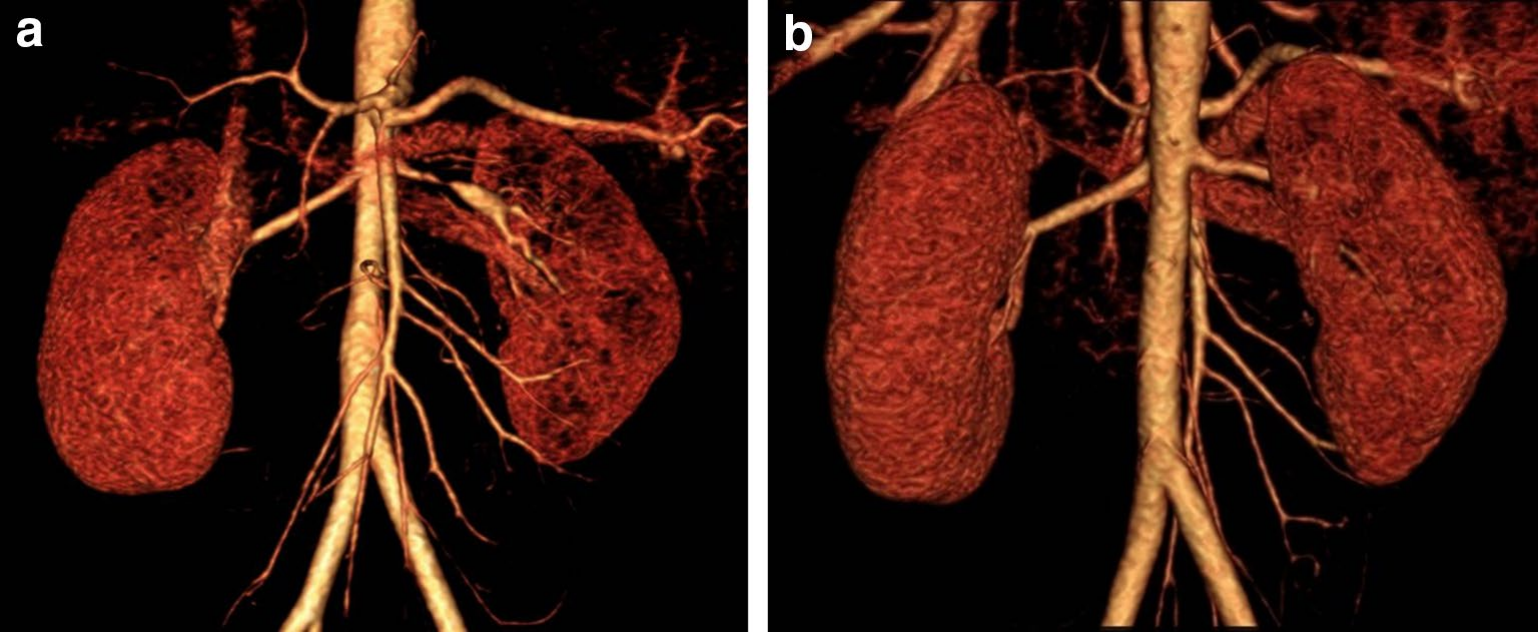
Three-dimensional multi-detector computed tomographic angiogram. a Severe focal stenosis is observed in the proximal area of the left renal artery on pre-mapping with 64-channel multi-detector computed tomographic angiography, before the interventional procedure. b Dilated and patent renal artery stenosis is observed at 7 months after cutting balloon angioplasty
